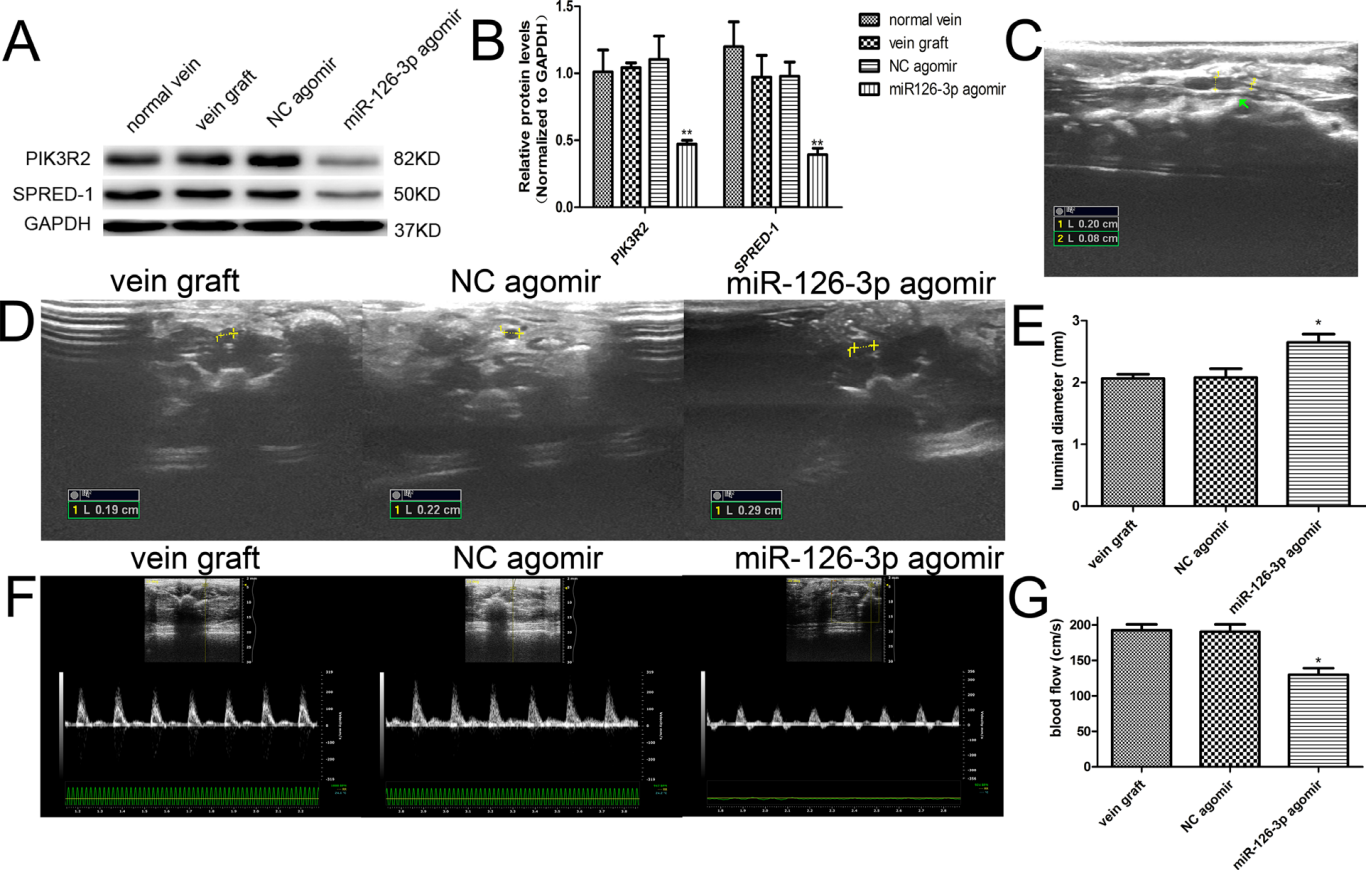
Exogenous miR-126-3p improved blood flow in vein grafts *in vivo* (A) Representative western blots and quantification (B) of PIK3R2 and SPRED-1 proteins are shown. PIK3R2 and SPRED-1 protein levels were significantly downregulated by local delivery of the miR-126-3p agomir at 7 days after transfection. (C) The vein grafts were all patent during the whole observation period, and longitudinal imaging emphasizes the internal diameter of the cannula and the vein graft at 4 weeks after surgery. (D) Representative ultrasound images and quantification (E) of the luminal diameter in vein grafts at 28 days after surgery are shown. (F) Representative ultrasound images and quantification (G) of the peak systolic velocity in vein grafts at 28 days after surgery are shown. Values are expressed as the mean ± SEM. *n =* 3–6 per group. P* < 0.05, *P* < 0.01 vs NC agomir and vein graft groups.
